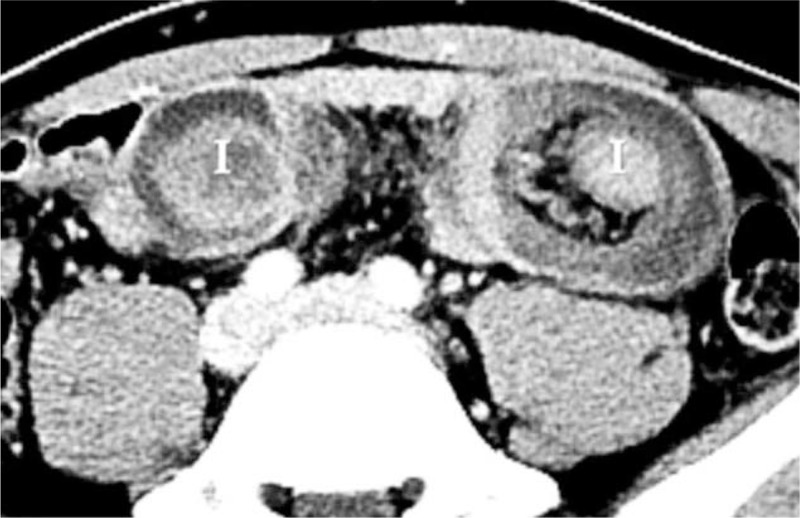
Axial section of computed tomography demonstrates duplicated layers of the bowel forming concentric rings, also called target sign. I = intussusception.
